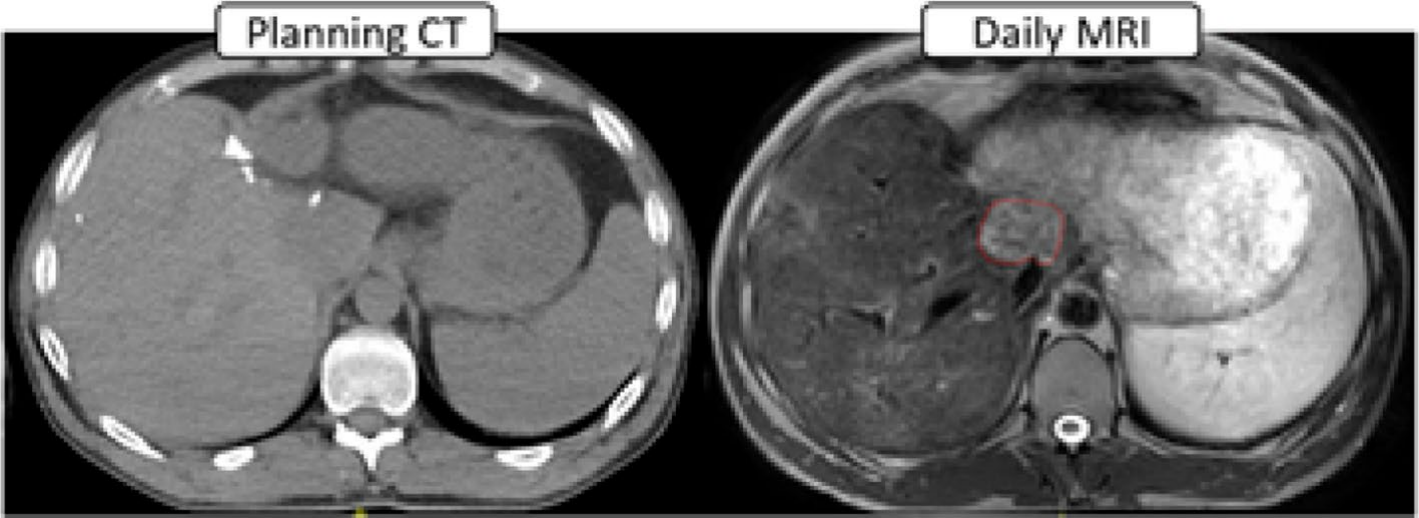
Abnormal tissue (circled on right) is more clearly visualised and defined on MRI than CT
